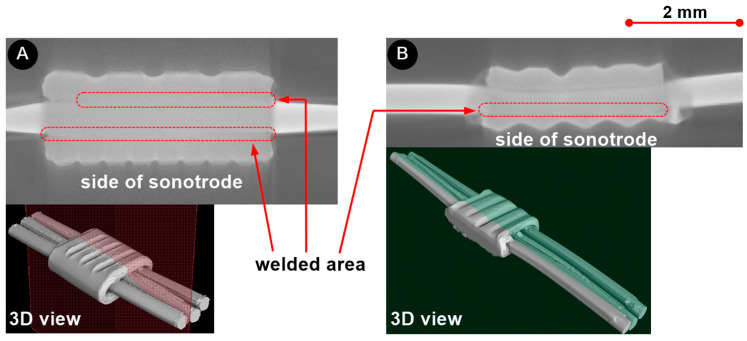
Analysis of CT scan of the UW joint (wire and ring) (A) without and (B) with varnish.
